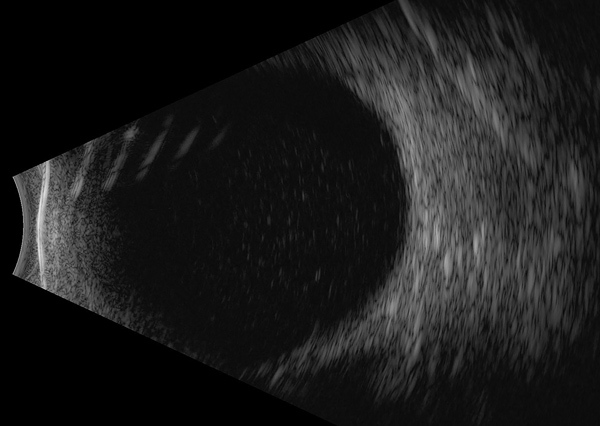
B-scan ultrasonography of the left eye of a 63-year-old woman with *Gordonia bronchialis*–associated endophthalmitis, Oregon, USA, showing dense opacities in the vitreous space.
